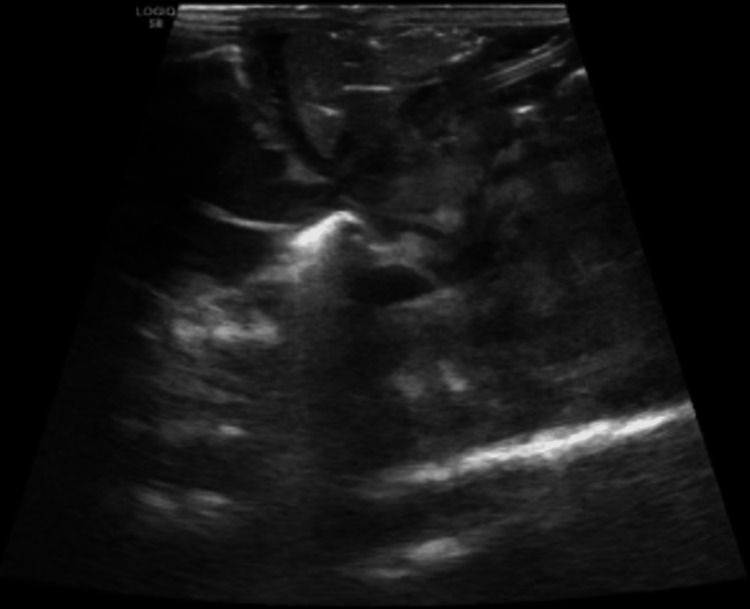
Abdominal ultrasound showing umbilical venous catheter (UVC) entering the left lobe of the liver, surrounded by edema.
